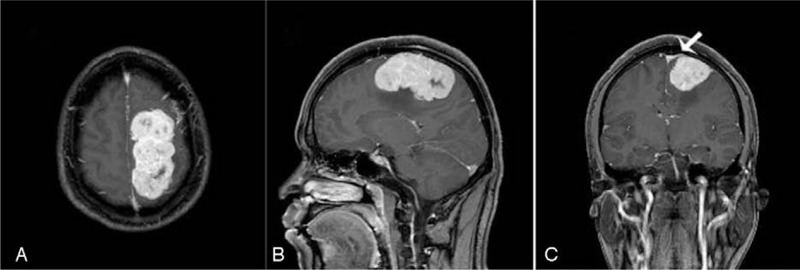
MRI revealed strong contrast enhancement on (A) the horizontal plane, (B) sagittal plane, and (C) frontal plane; “dural tail” sign can be seen in the frontal plane. MRI = magnetic resonance imaging.
